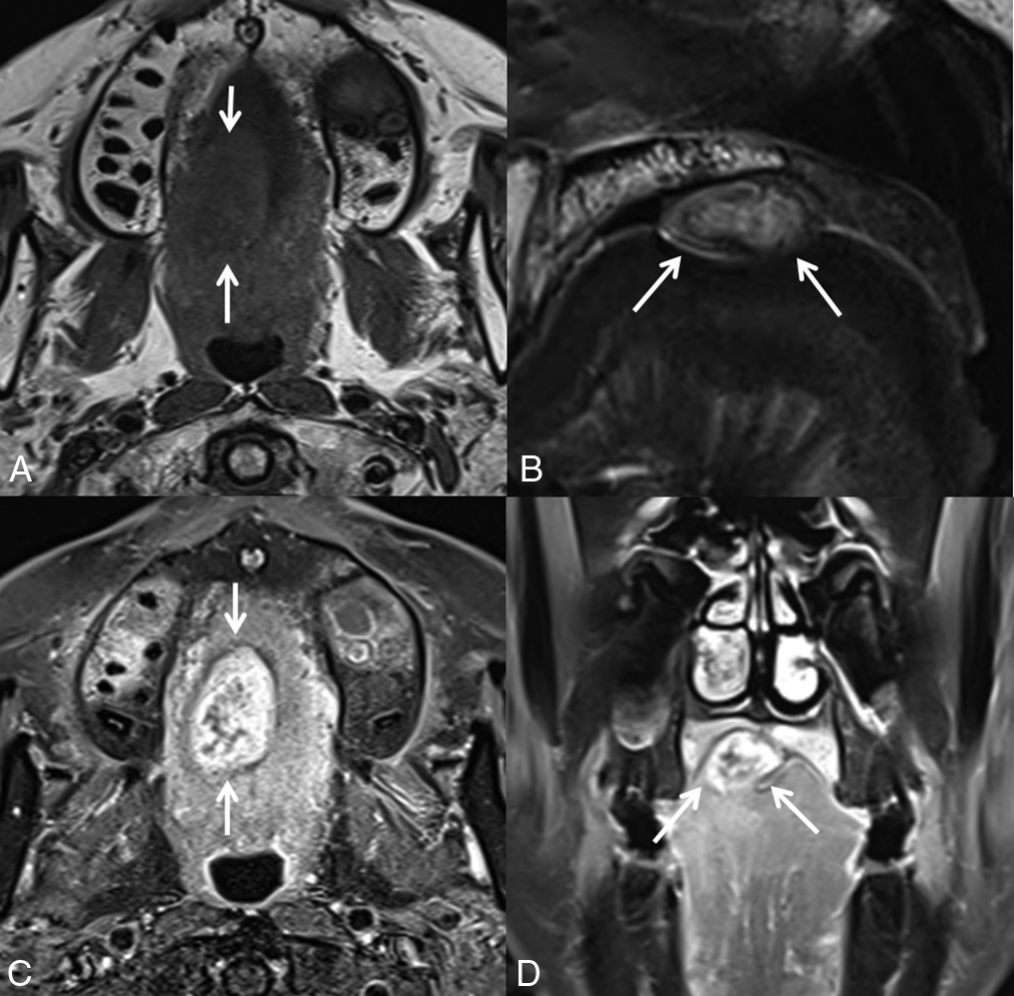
On contrast-enhanced oropharyngeal magnetic resonance imaging (MRI): (A) the oval-shaped lobulated mass with intermediate signal intensity on T1-weighted images, and (B) heterogeneous high signal intensity on T2-weighted images. (C, D) The lobulated mass showed a heterogeneously enhancement crossing the midline.
